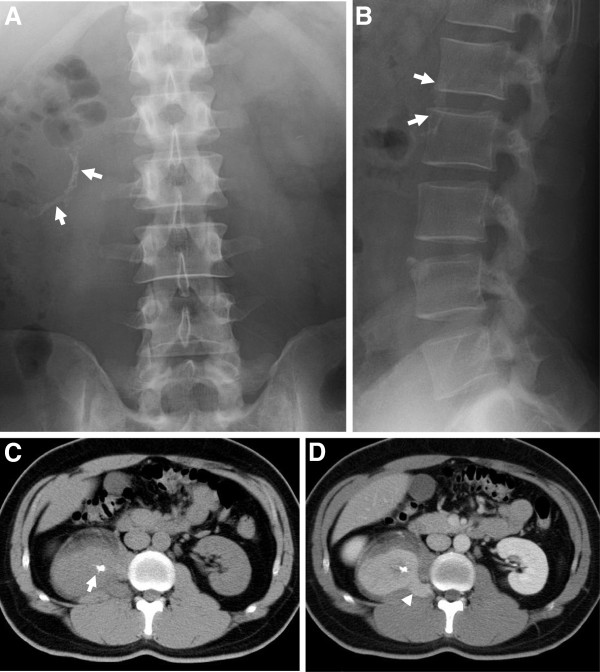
Plain radiography and computed tomography. A striated calcification on the right side of the first and second lumbar vertebrates was noted (arrow) in abdominal plain radiography (A,B). Computed tomography revealed a homogenous mass with central calcification (arrow) in the swollen psoas muscle (C). After contrast enhancement, the mass presented a dumbbell-like shape, extending to the intervertebral foramen (D).
